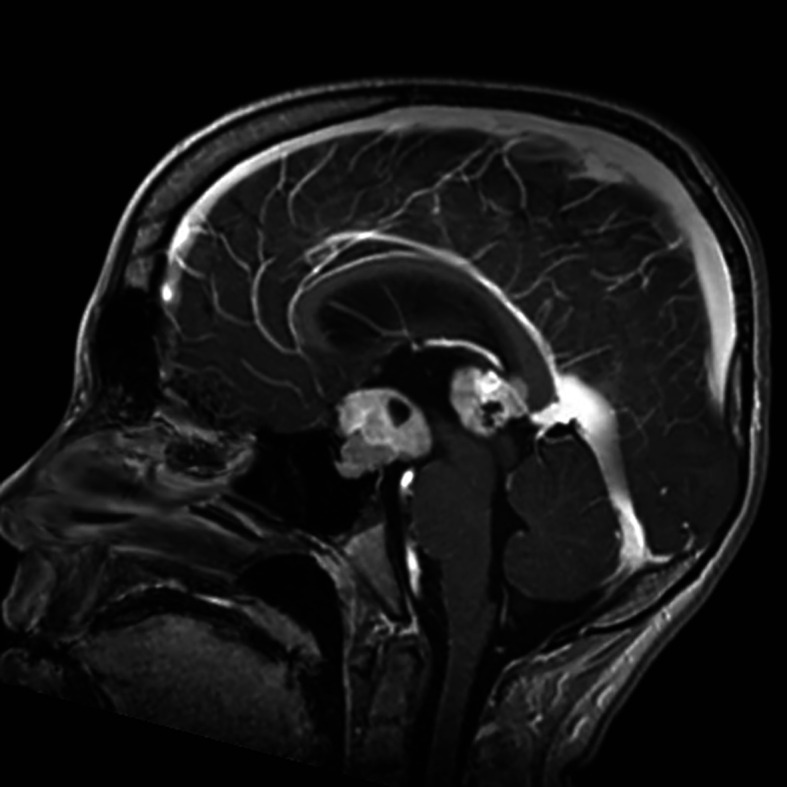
Post-contrast sagittal T1-weighted MRI showing bifocal germinoma.
